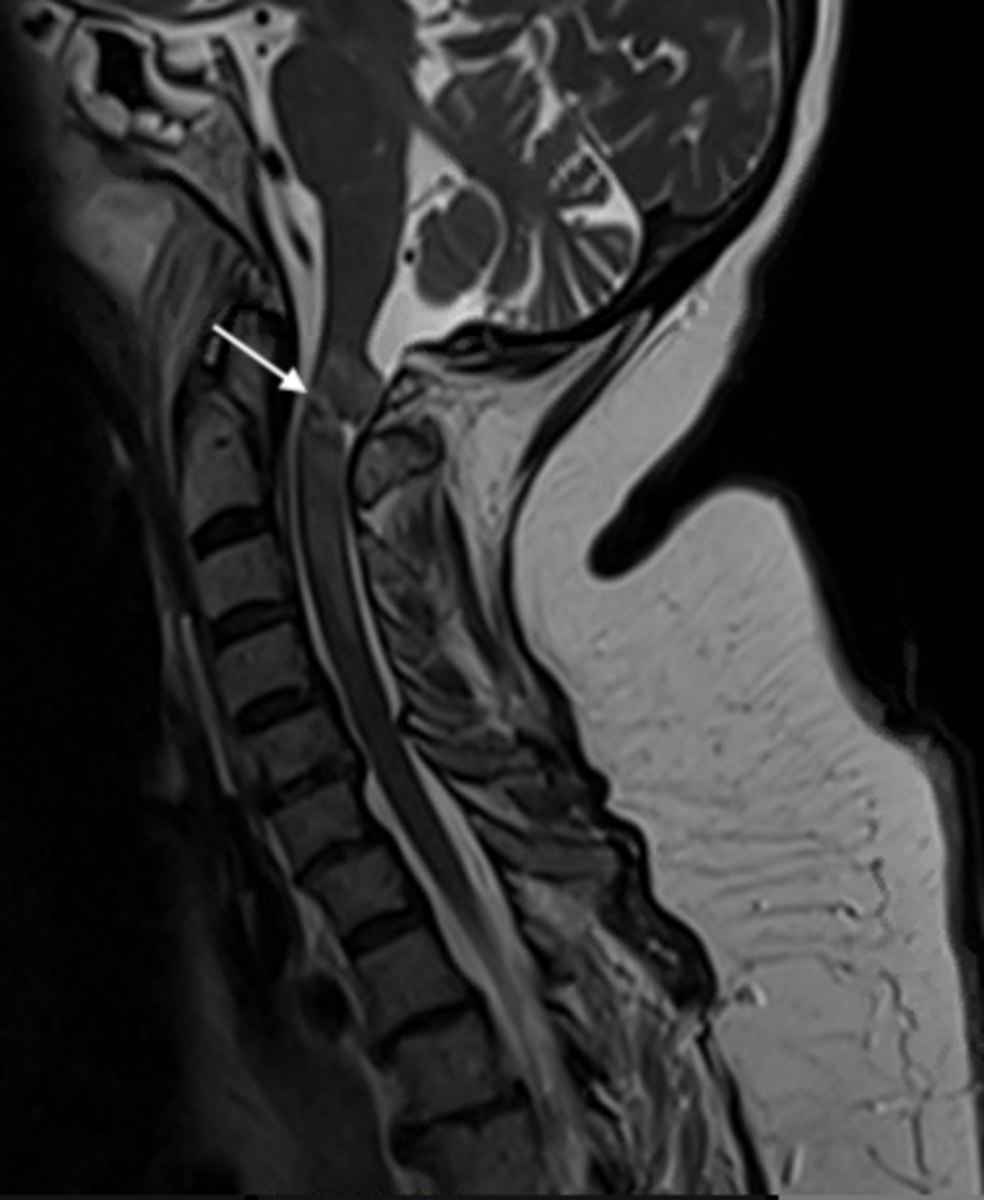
T2-weighted MRI of the cervical spine with a parasagittal view. The parasagittal view demonstrates high-grade partial spinal cord transection at the level of C1-C2. There is also an associated spinal cord contusion and a small amount of intrathecal blood.
